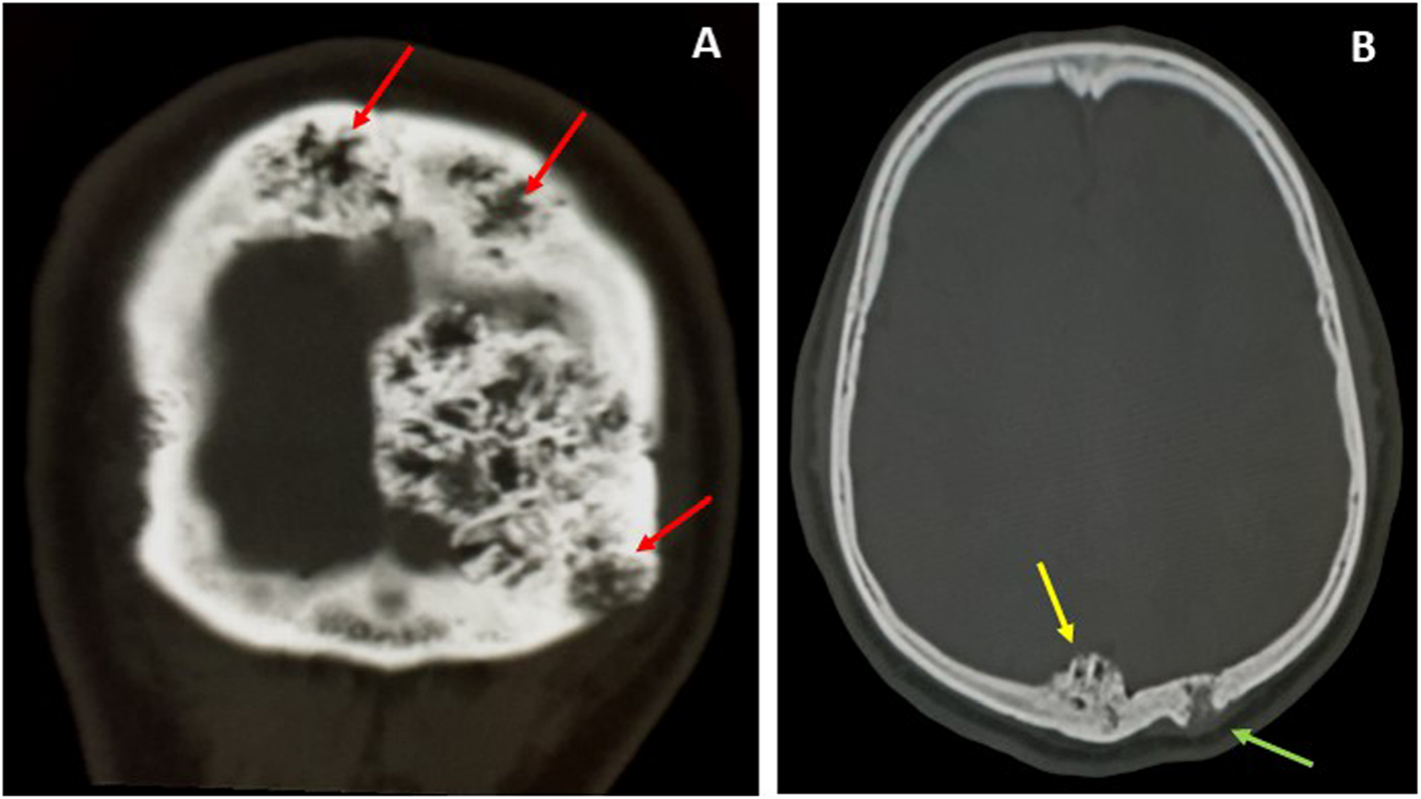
Computed tomography scan bone window coronal image showed 3 variables sized, rounded, diploic, expansile, lytic lesions with sunburst appearance (Red arrows), two biparietal, and left occipital (a). CT scan bone window axial image showed biparietal diploic lesions (b). The right one without wall erosion (Yellow arrow) while the left one with external wall erosion and subgaleal soft tissue (Green arrow)
